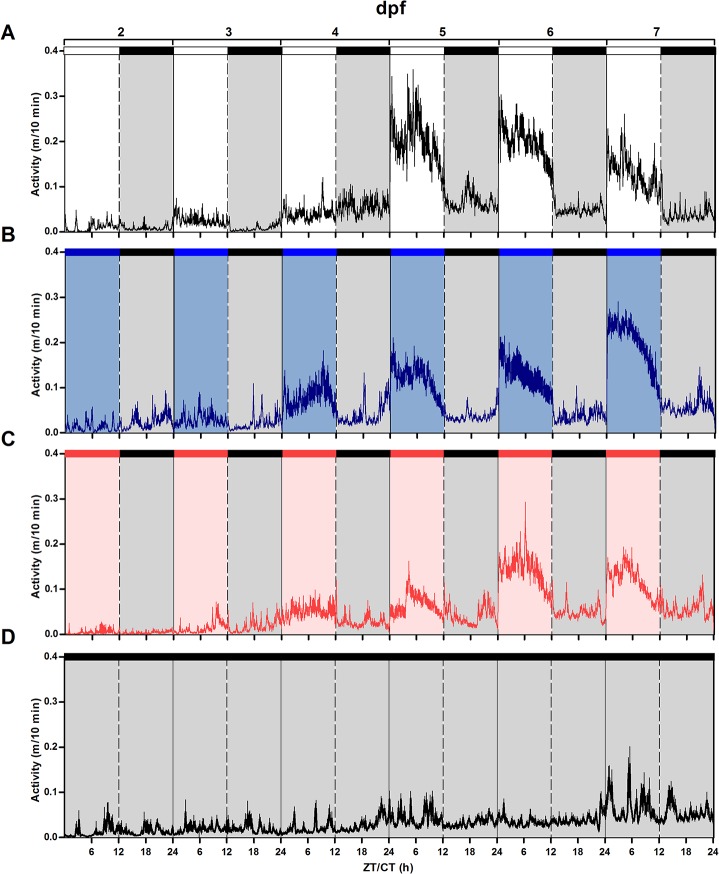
Mean waveforms of locomotor activity under different lighting conditions from 2 to 7 dpf. Larvae reared under different light conditions (A: LDW, n = 35, B: LDB, n = 30, C: LDR, n = 30, D: DD, n = 45). Vertical axis represents activity (m/10 min) and horizontal axis zeitgeber/circadian time (ZT/CT). Bars above each panel indicate the lighting conditions [black bars indicate darkness, white bars indicate white light (LDW), blue bars indicate blue light (LDB), and red bars indicate red light (LDR)] and the day post-fertilization (dpf). Data are expressed as mean ± SEM.
